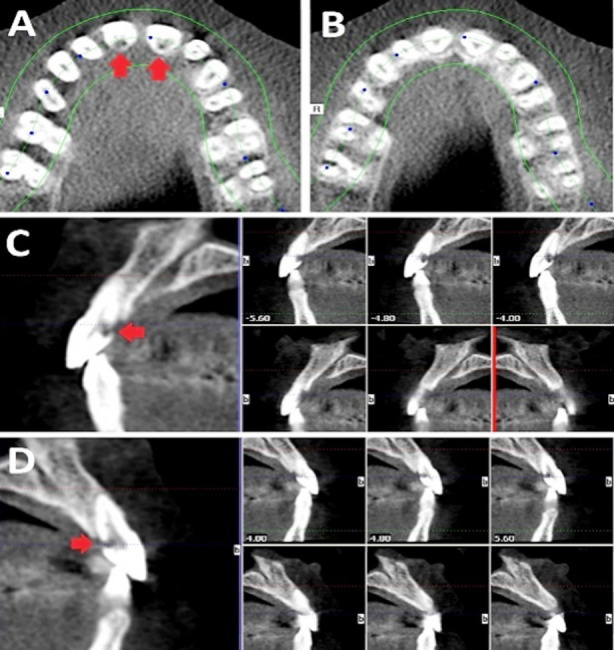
Pre-operative CBCT image, red arrows showing the location of cervical resorption (A) Transverse section at cervical region, (B) Transverse section at mid-root region, (C) Sagittal sections of tooth #11, (D) Sagittal sections of tooth #21.
